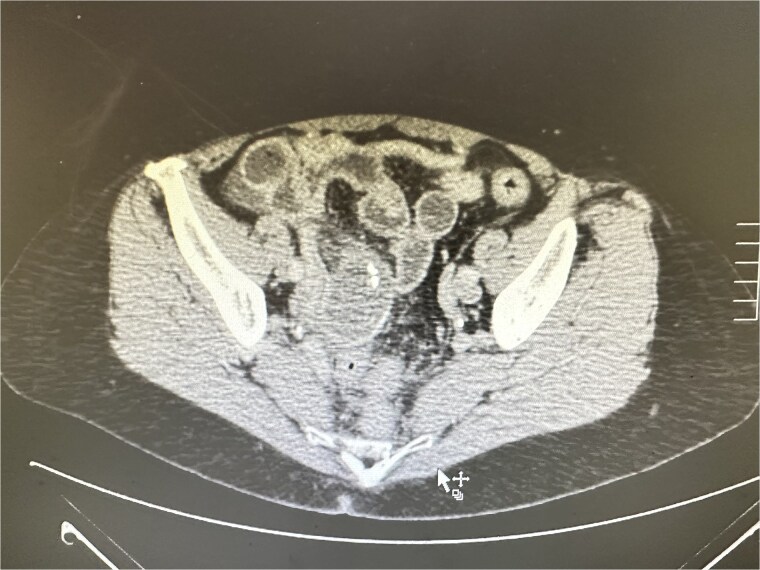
CT revealed a large predominantly cystic mass posterior to the uterus with mural calcifications and apparent continuity with the appendix, indicating an appendiceal mucocele.
